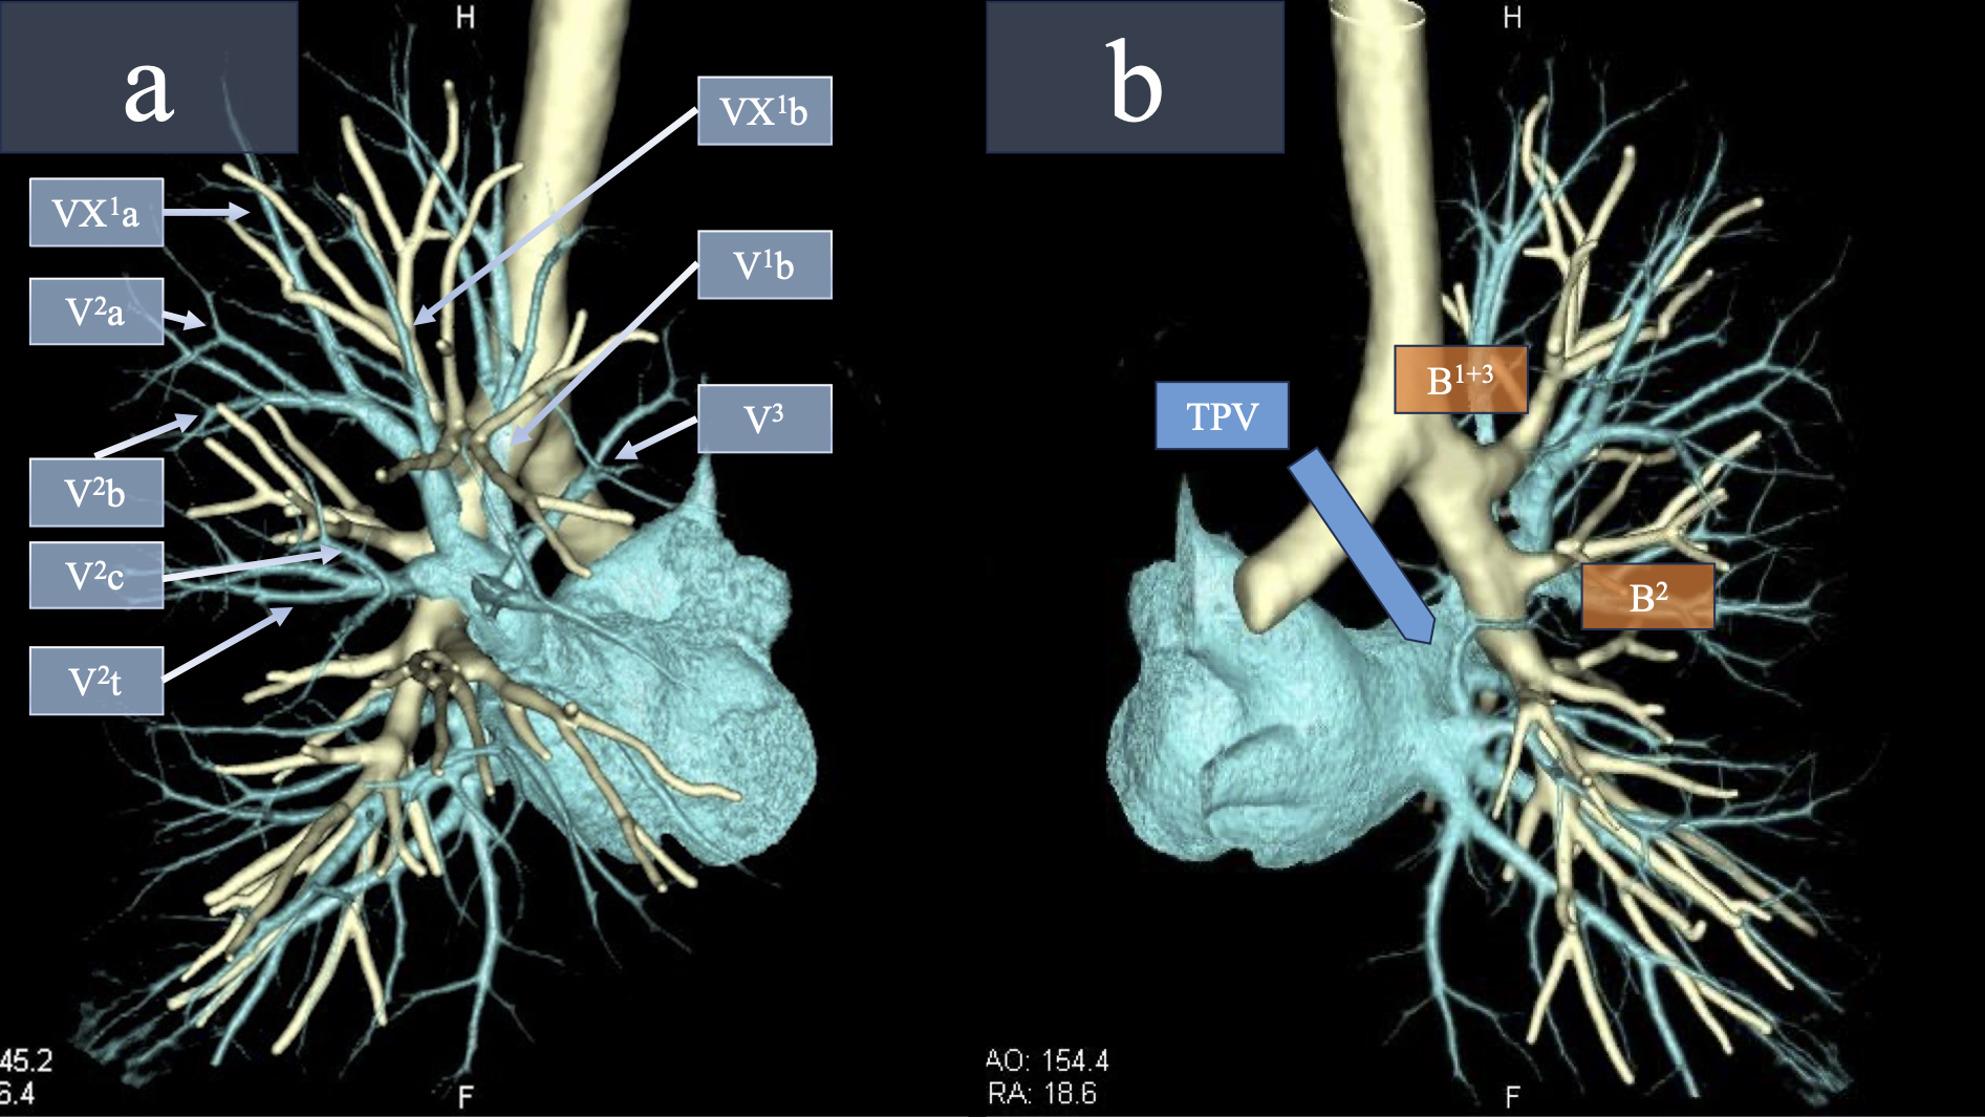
Three-dimensional CT bronchography and angiography (3D-CTBA), venous phase, for preoperative anatomical planning (Ziostation REVORAS; Ziosoft Inc., Tokyo, Japan). a In addition to V1b, two veins from segment S1 (VX1a and VX1b) drained into the central vein. b The top pulmonary vein was formed by a portion of V2 draining into V6
